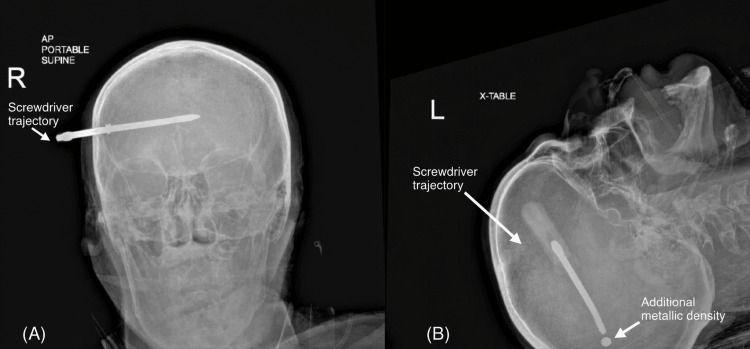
Skull radiographs demonstrating retained screwdriver and additional metallic density (A) Anteroposterior skull radiograph demonstrating the retained screwdriver traversing the cranial vault. (B) Lateral skull radiograph demonstrating the oblique depth of penetration and an additional metallic density near the distal end of the penetrating object, initially concerning for a detached screwdriver component and later clarified on CT as a retained bullet fragment from prior injury
